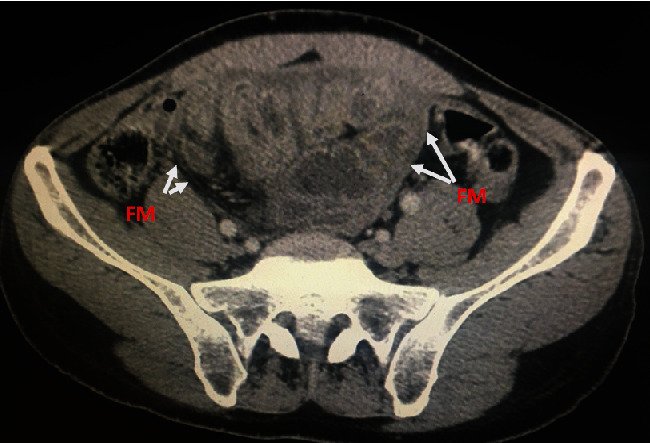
CT imaging dilated small bowel loops, encapsulated by a fibrous membrane (FM) and trapped in the right peritoneal cavity.
